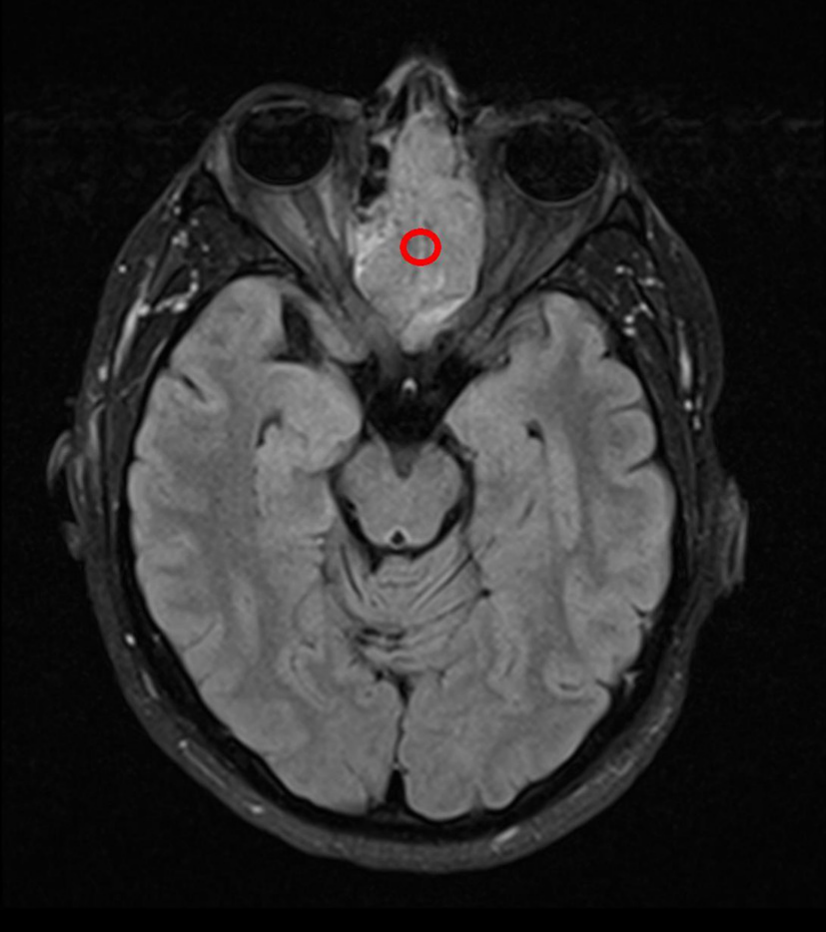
Axial *T*_2_ weighted turbo inversion recovery magnitude MRI showing a lobulated soft tissue mass (red circle) expanding the nasal septum bilaterally with slight hyperintense signal. Some hypointensities, possible flow voids, are seen.
